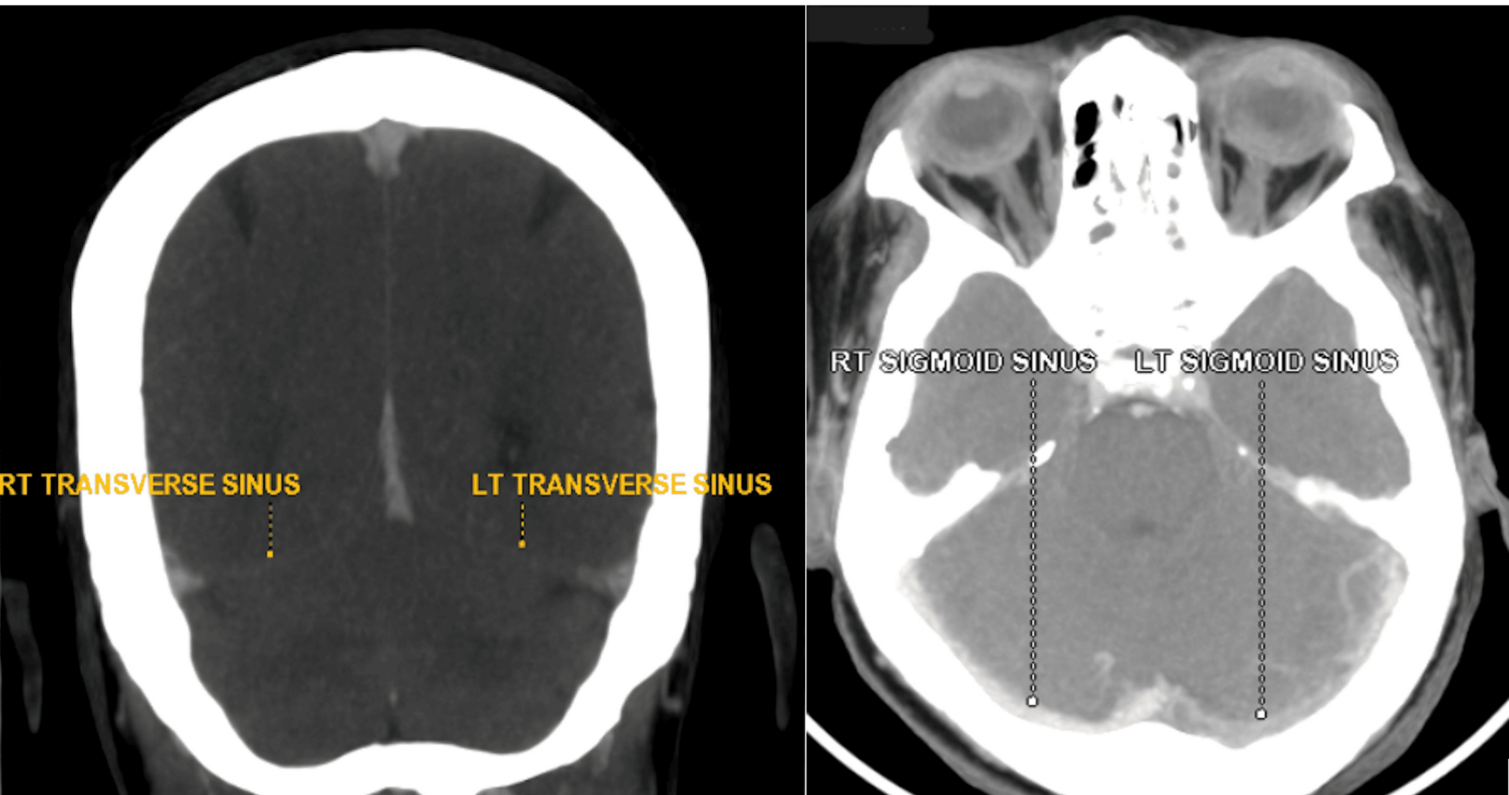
CT cerebral venogram showing partial thrombosis in the left sigmoid sinus and left transverse sinus.
